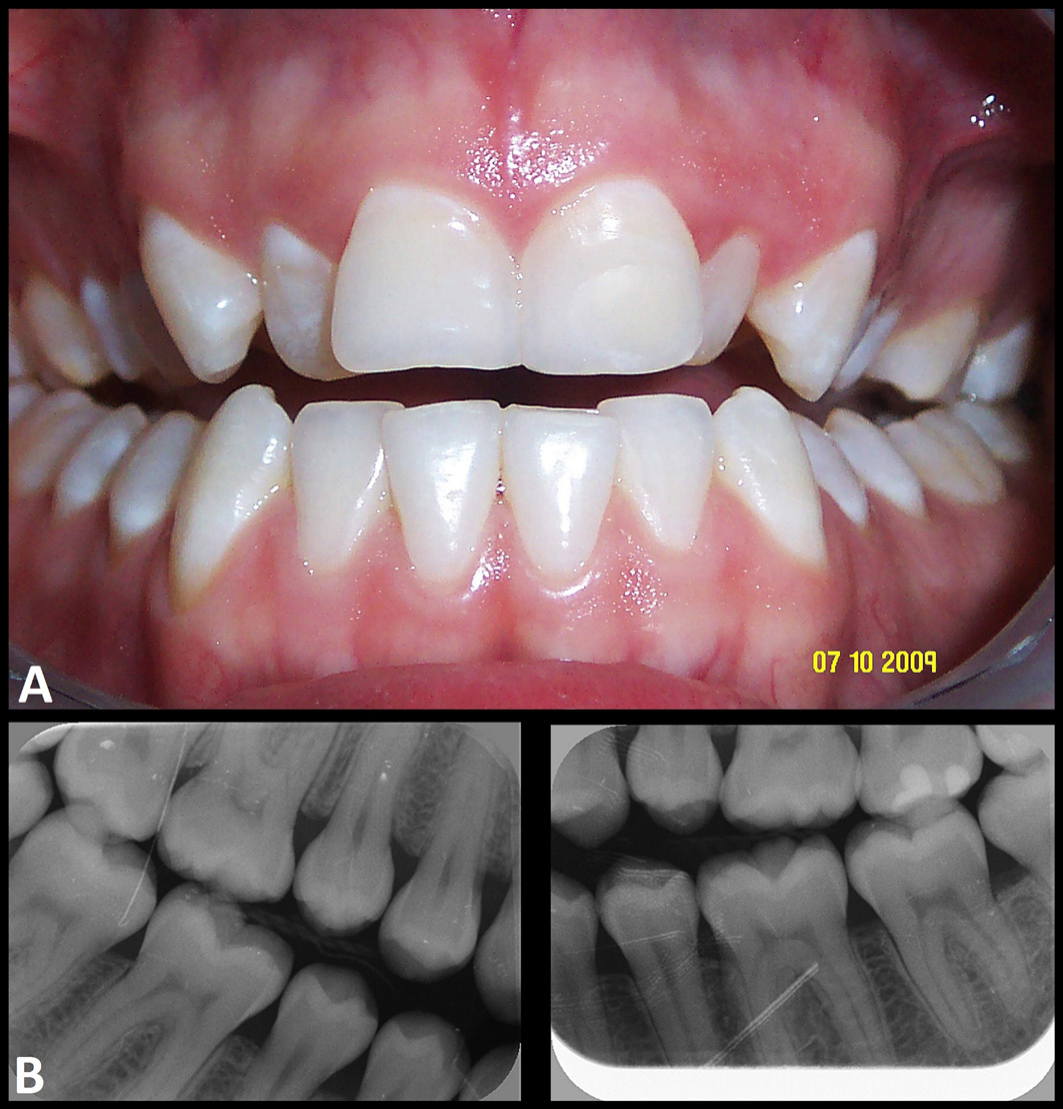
Images of the patient in 2009, at 21 years of age. A. Dental occlusion. B. Bite-wing radiographic views.
